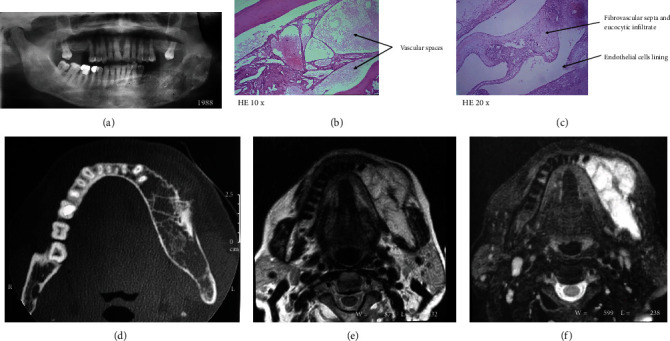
(a) Panoramic radiograph showing a well-defined multilocular radiolucency extending from the mental nerve region to the ramus. (b) Thin-walled and dilated vascular spaces of variable diameter (HE ×10). (c) Regular endothelial lining of the vascular spaces with mild inflammatory polymorphous leucocytic infiltrates in the surrounding tissue (HE ×20). (d) An axial CT image showing an expansible multilocular radiolucency lesion with periosteal reaction on the buccal cortex. (e) An axial T2WI MR image revealing a hyposignal intensity expansible low-intensity lesion in the marrow of the left hemimandible with cortical rupture and soft tissue extension. (f) An axial T2 WI FSE image presented a hypersignal and expansible lesion with internal loculations suggesting a multilocular lesion.
